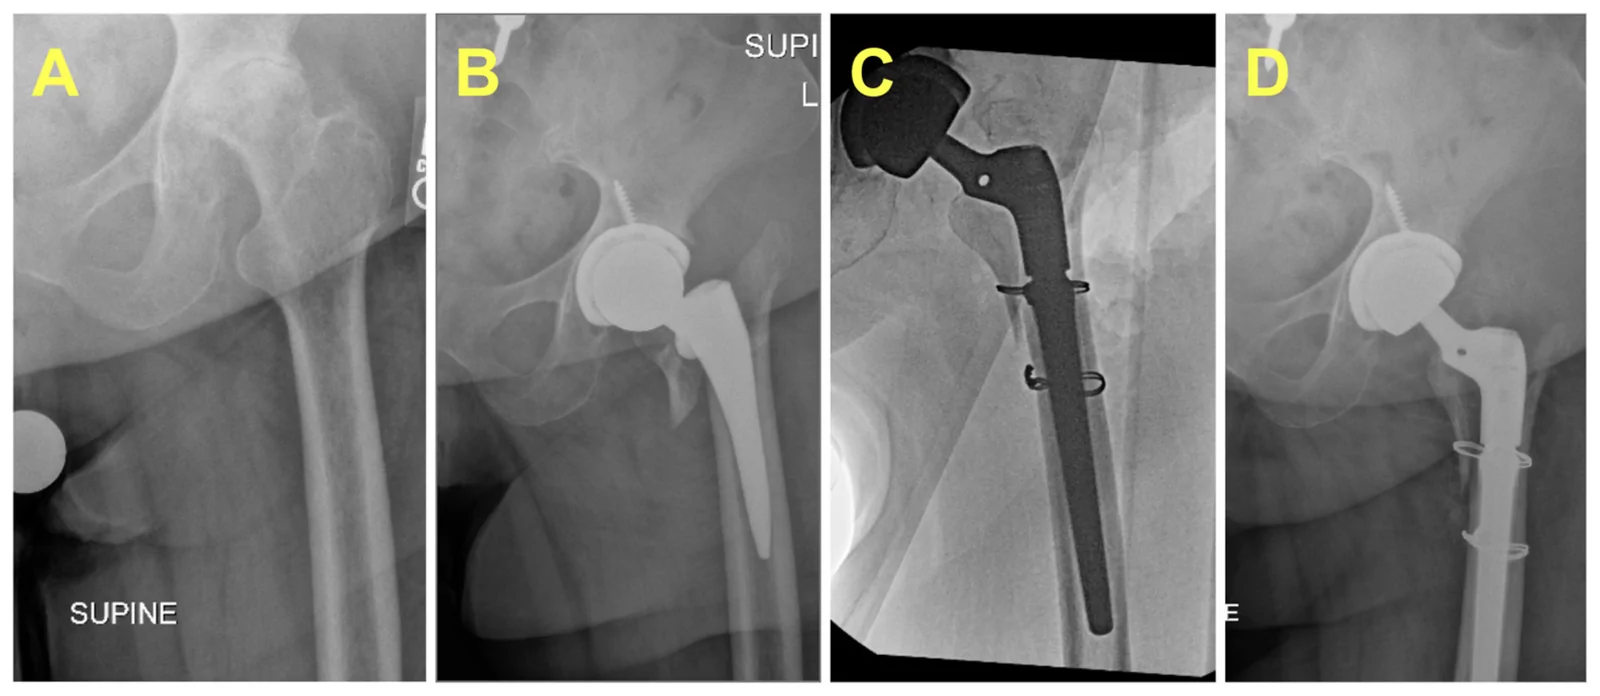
Preoperative (A) and postoperative (B–D) radiographs demonstrating a periprosthetic Vancouver B2 fracture. The patient began feeling pain overnight on postoperative day 15. They reported to the emergency department, and a fracture was found (B). The stem was revised, and two cables were placed (C). A 4-week postoperative radiograph following revision (D).
